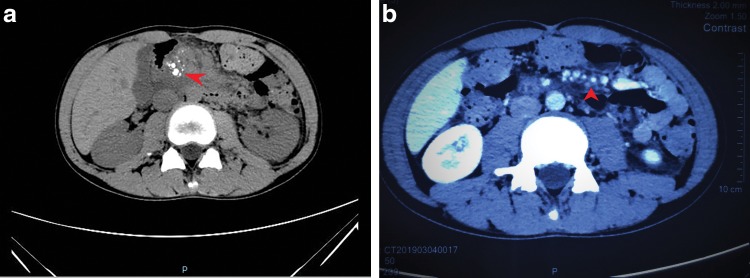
(a, b) Abdominal CT showing pancreatic duct stones in the body of pancreas with multiple high density calcifications in the head of pancreas. Red arrow: stones and pancreatic head calcification (a); pancreatic duct stones (b). CT, computed topography.
